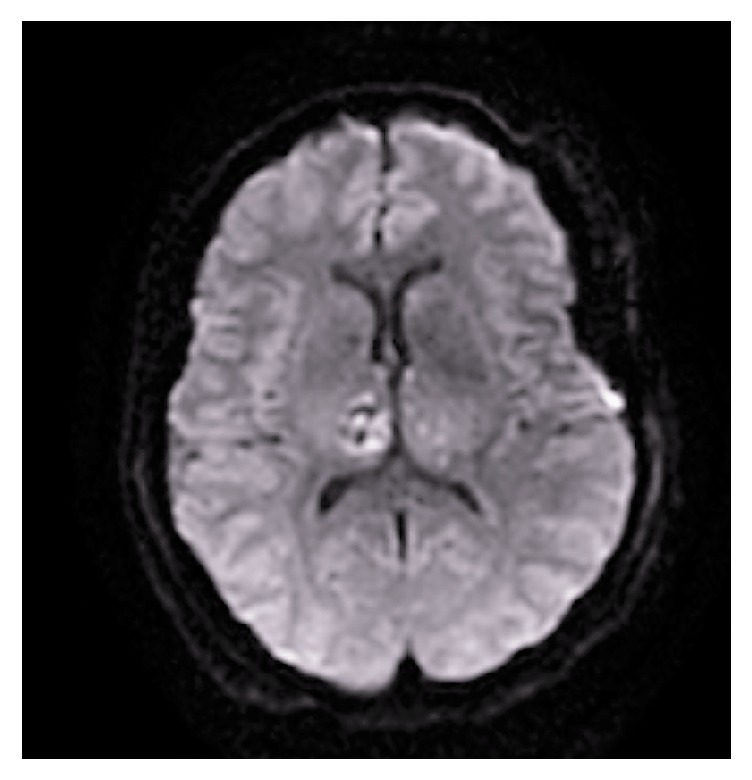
Diffusion weighted image MRI from postoperative day 1.* Note*. Increased signal intensity in right basal ganglia with punctate increases on the left indicating acute infarcts.
